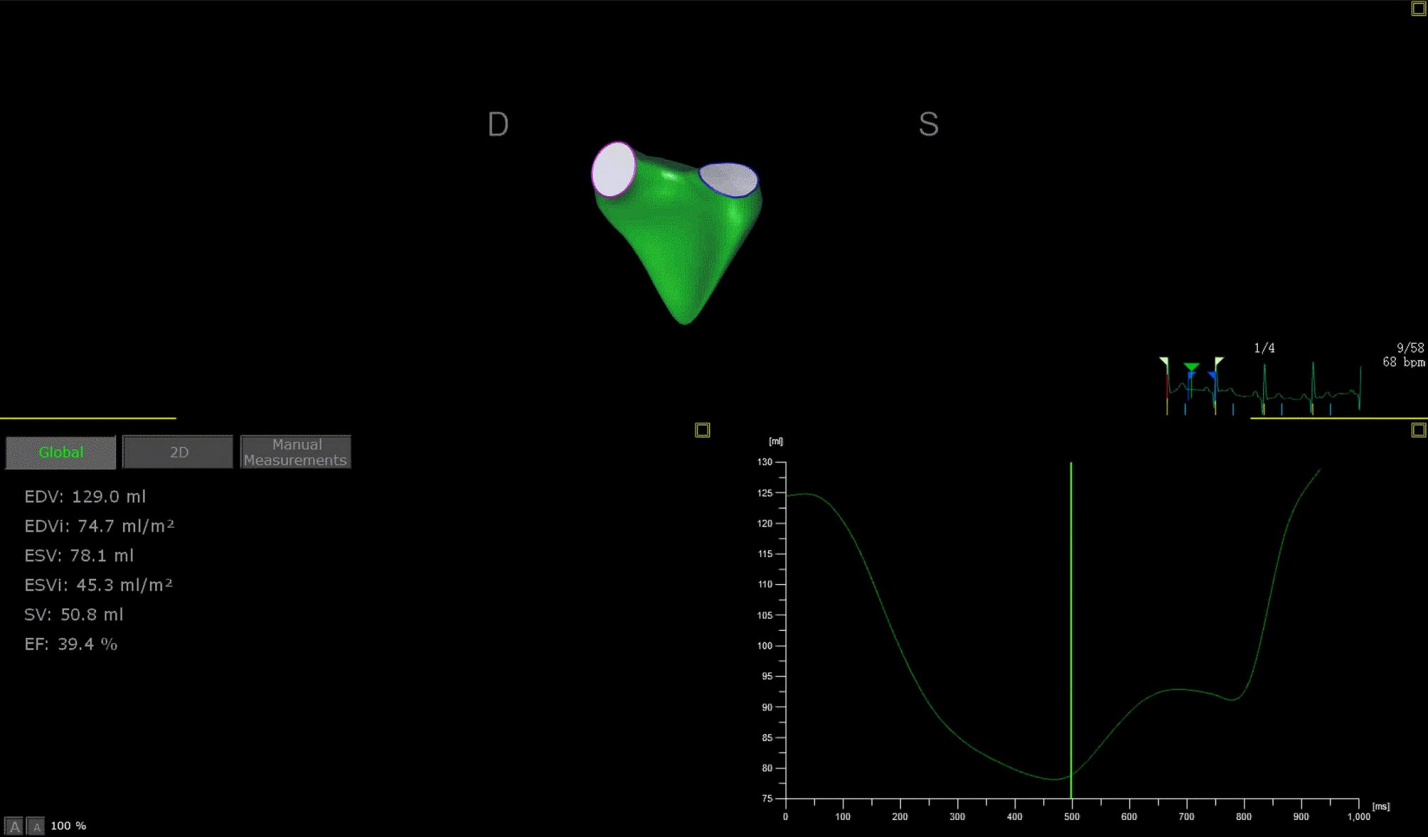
An example of OSAS patient for calculation of right ventricular end-diastolic and end-systolic volumes utilizing three-dimensional echocardiography.
